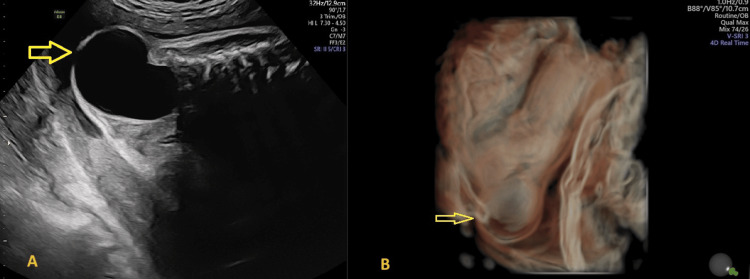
Ultrasound (sagittal view) at 35 weeks of (A) the progressively enlarging pelvic mass (arrrow), and (B) the corresponding 3D reconstruction (arrow) 3D: three-dimensional
